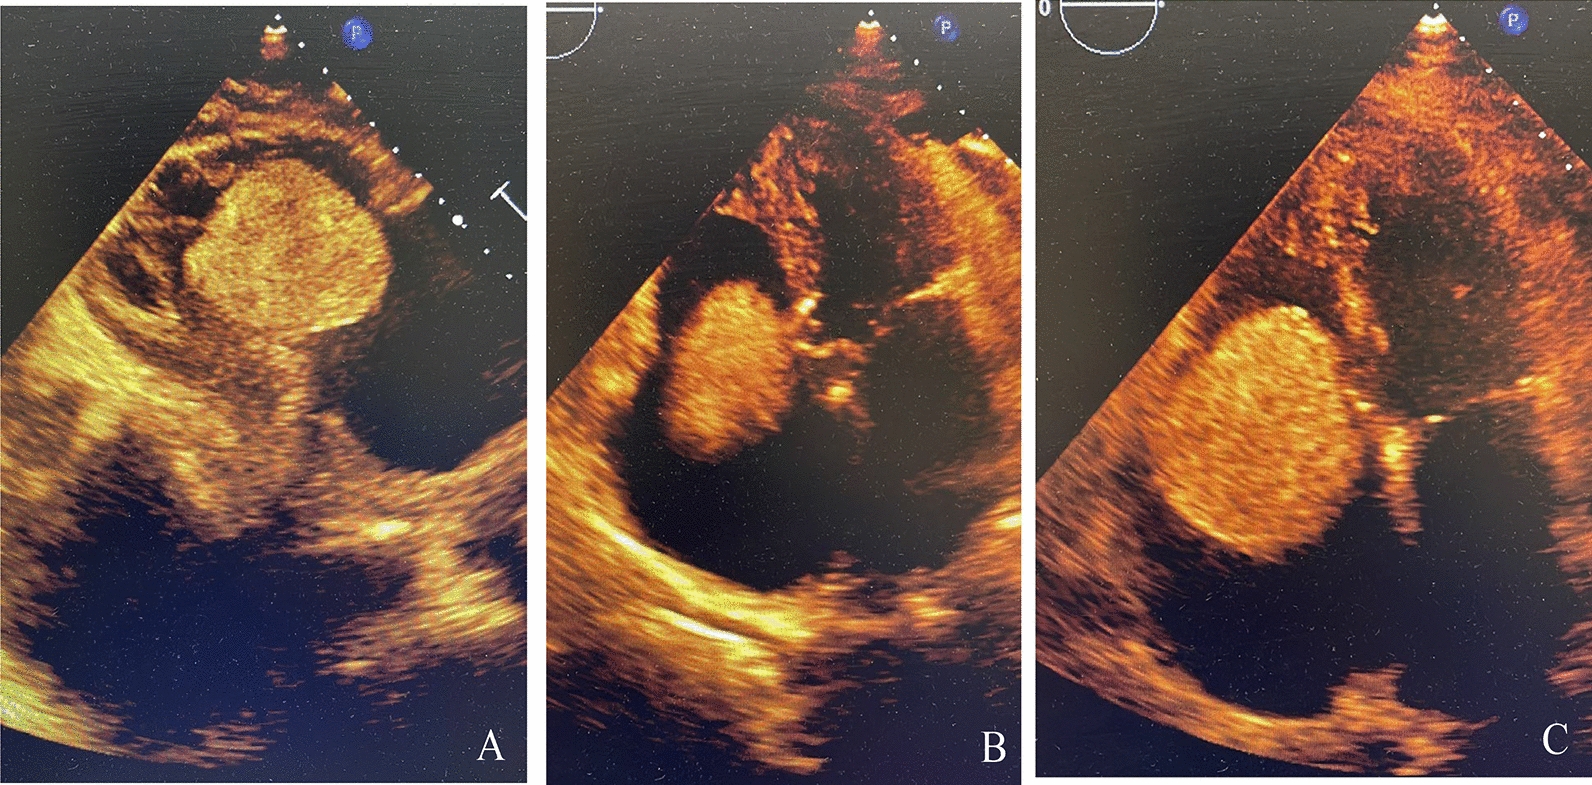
Transthoracic echocardiography of the mass in the right chambers. A mass obstructing the tricuspid valve, subcostal view. B mass moving back and forth through the tricuspid valve in apical 4 chambers view. C Systolic tricuspid valve obstruction in apical 4 chambers view
